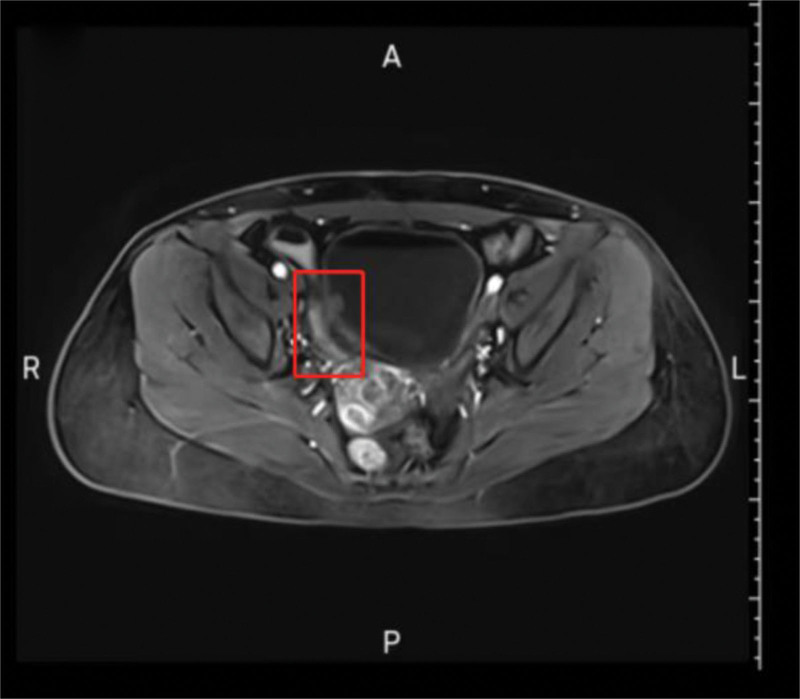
Preoperative pelvic MRI (T2-weighted image) showing abnormal signal at the right posterolateral bladder wall (arrow) with extraluminal tract to ileum. MRI = magnetic resonance imaging.
